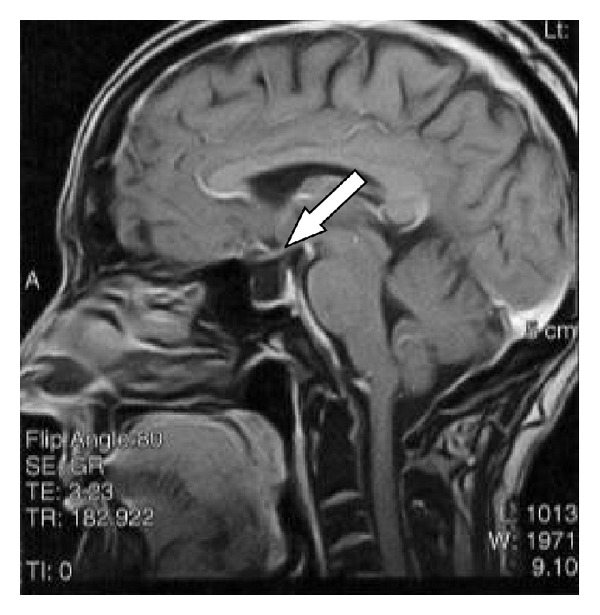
Partial empty sella syndrome with suprasellar cistern hernia on MRI.
